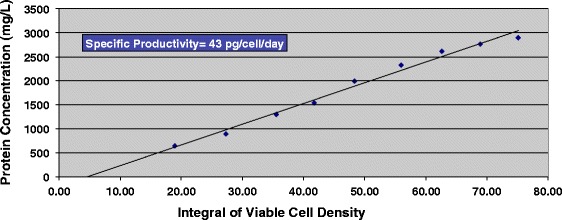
A GPEx® clonal cell line expressing CT-tagged antibody afforded high fGly conversion and high titers in a 100 L bioreactor production run. Cells were cultured in HyCell™ media supplemented with 50 μM copper(II) sulfate. The protein concentration and integral of viable cell density is shown. Cultures were terminated when viabilities were ≤ 80 %. Titers of 2.9 g/L were reached. The cells demonstrated a specific productivity of 43 pg/cell/d, and the resulting antibody had fGly conversion yields of 96.4 %
